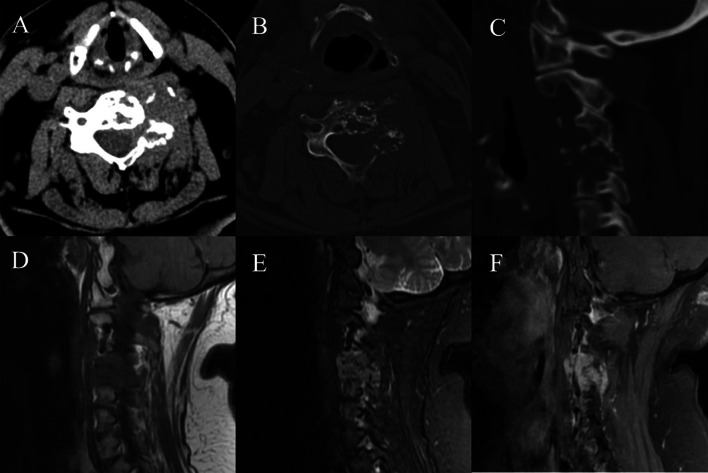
A 73-year-old female with C4 primary leiomyosarcoma. A Axial computed tomography (CT) showed an ill-defined isodense mass in the vertebral body and posterior elements of C4; B axial CT bone window showed osteolytic bone destruction, with residual bone trabeculae; C sagittal CT bone window showed osteolytic bone destruction; D T1 weighted imaging (T1WI) in sagittal view showed an isointense mass; E T2 weighted imaging (T2WI) in sagittal view showed an isointense mass; F Magnetic resonance imaging (MRI) enhanced scanning showed homogeneous enhancement of the mass that appeared lobulated
